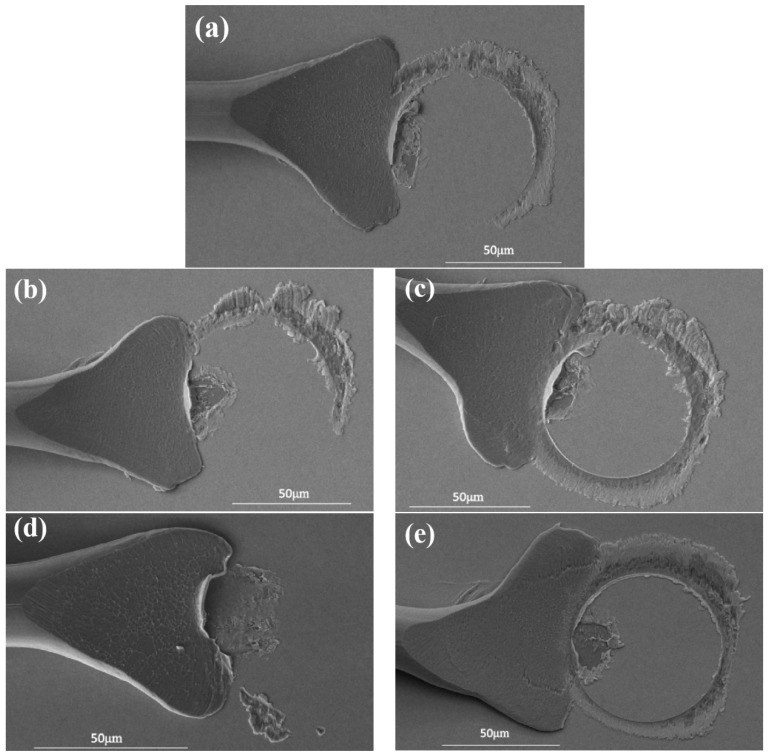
Morphology of Pd120 wedge-bonded points under different bonding pressures/ultrasonic power ((a) 55 g/115 mW; (b) 55 g/105 mW; (c) 55 g/125 mW; (d) 50 g/115 mW; (e) 60 g/115 mW).
